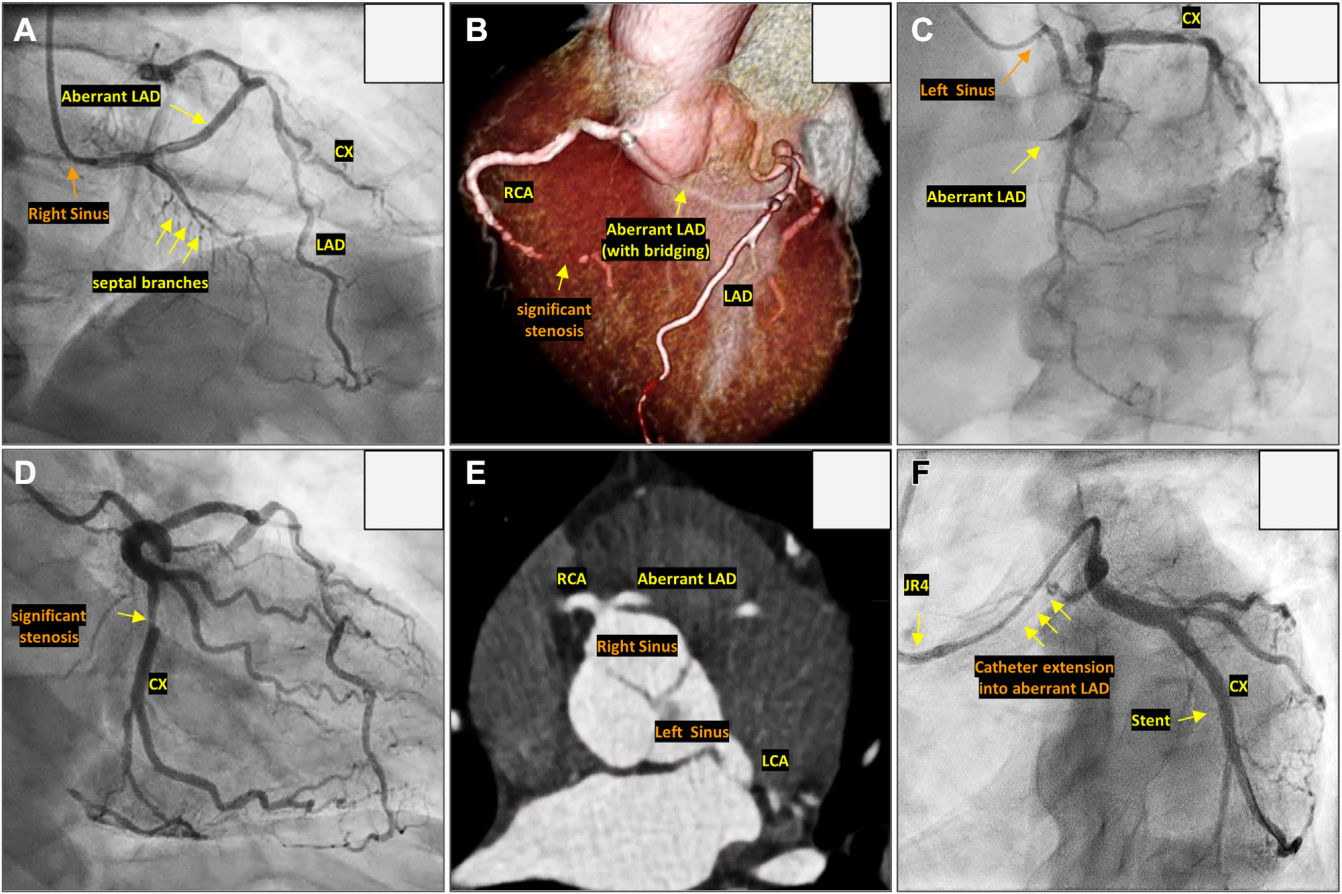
(A) Coronary angiography showing an aberrant left coronary artery (LCA) arising from the right coronary sinus; (B) 3D coronary computed tomography scan showing the aberrant LCA originating from the right coronary sinus with an intramyocardial course; (C) coronary angiography from the left coronary sinus revealing competing flow between the aberrant and native LCA toward the mid-distal left anterior descending (LAD) artery; (D) coronary angiography showing significant mid-circumflex artery (CX) stenosis; (E) computed tomography scan showing the dual origin of the LCA; and (F) final result of percutaneous coronary intervention of the CX. JR4, Judkins right 4; RCA, right coronary artery.
